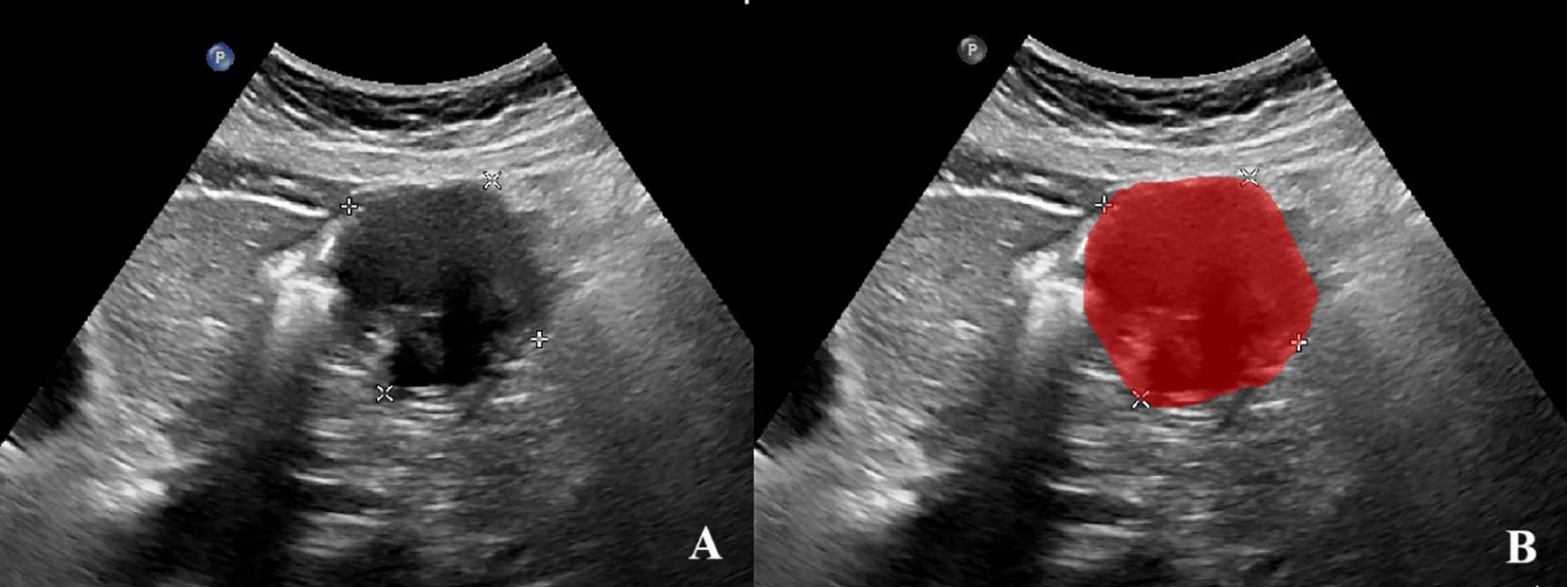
Annotation of the tumor ROIs. (A)The GIST ultrasound image based on oral contrast-enhanced ultrasonography. (B)The red region indicates the region of interest (ROI).
